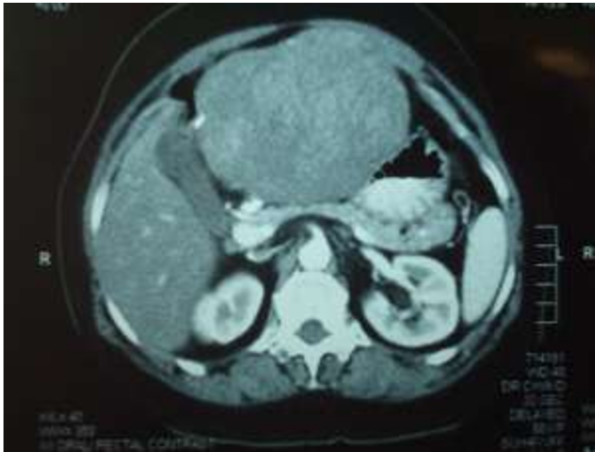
CT Scan abdomen showing the mass.
